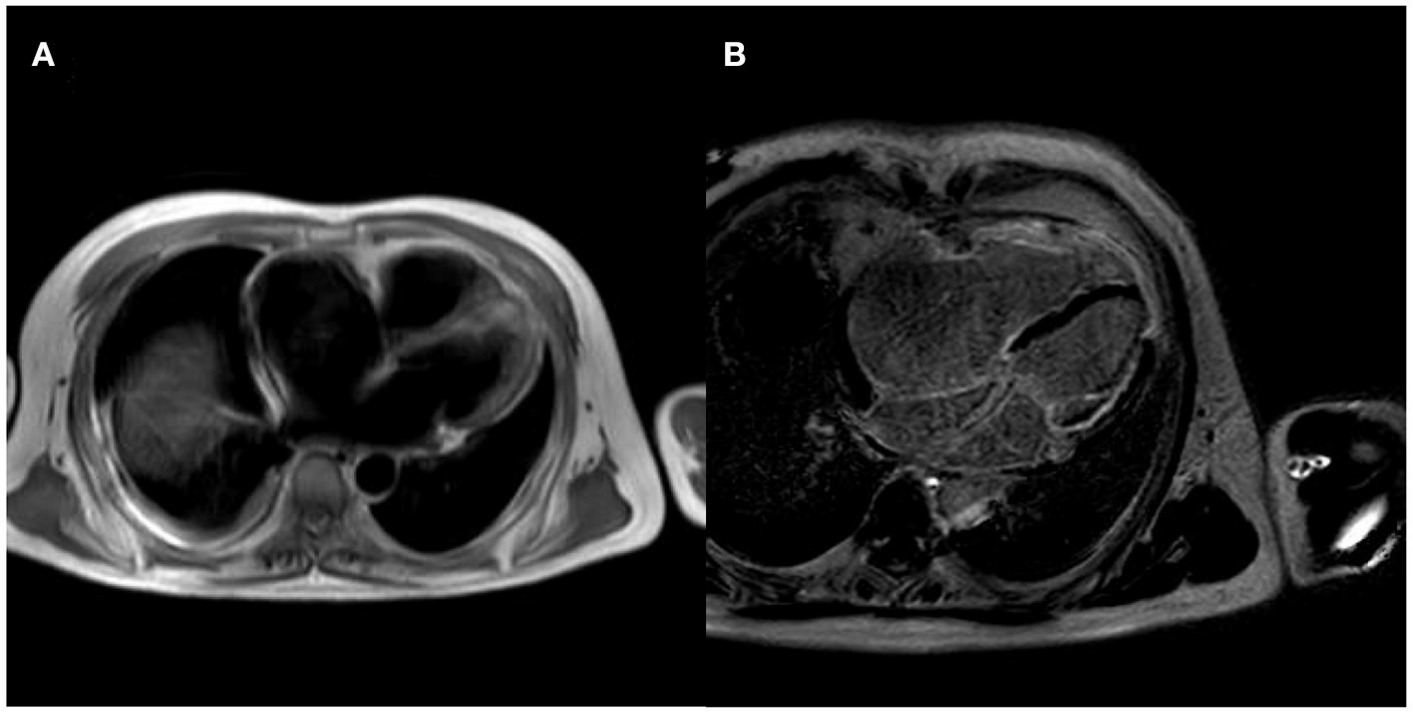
MRI of the patient's heart [(A) is a whole heart cross-sectional view, which shows a small left atrium and an enlarged right atrium; (B) is a delayed scan, which shows diffuse strip enhancement of the left ventricular lateral wall, apical region, and right ventricular wall, with some transmural-like enhancement].
